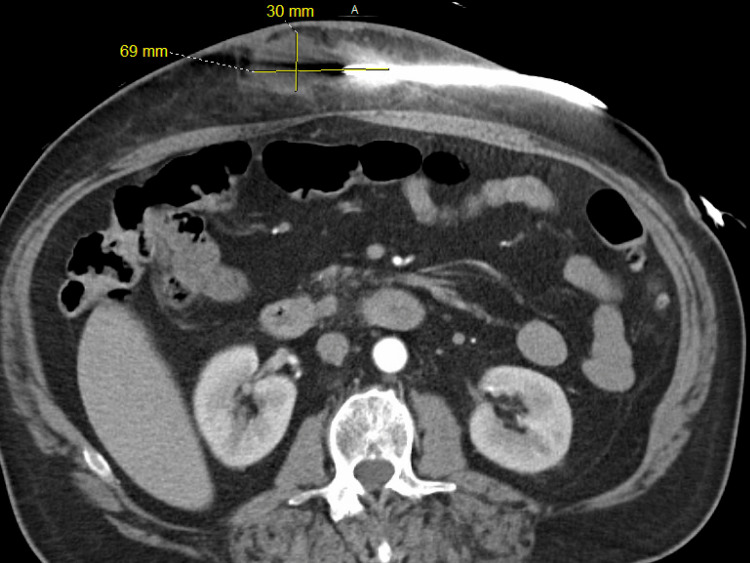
Computed tomography scan of the chest and abdomen during the initial evaluation of the LVAD driveline site shows a fluid collection (yellow lines) in the driveline site, indicating abscess formation. LVAD: left ventricular assist devices.
